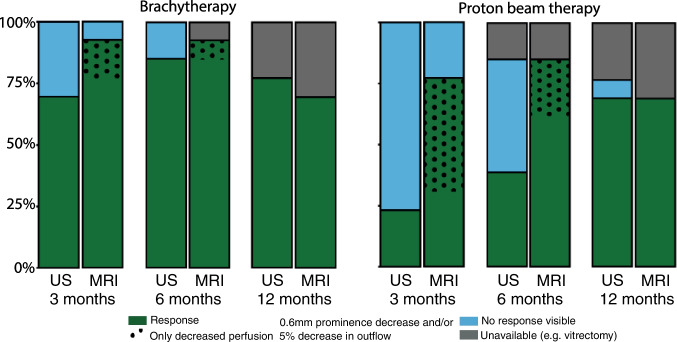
A side-by-side comparison of treatment response of (left) brachytherapy and (right) PBT-treated patients, based on MRI and ultrasound at each timepoint. Treatment response in terms of tumour prominence was defined as a decrease in tumour prominence of more than 0.6 mm. In addition, a reduction in outflow percentage of at least 5% on PWI was also considered a sign of treatment response on MRI. At 3 months, 69% of brachytherapy patients showed treatment response on ultrasound as compared to 92% patients on MRI. For PBT patients, however, only 23% of patients showed treatment response on ultrasound while this was 77% on MRI. Furthermore, 60% of those patients who showed treatment response on MRI were based on favourable PWI changes, when no prominence decrease was yet apparent
